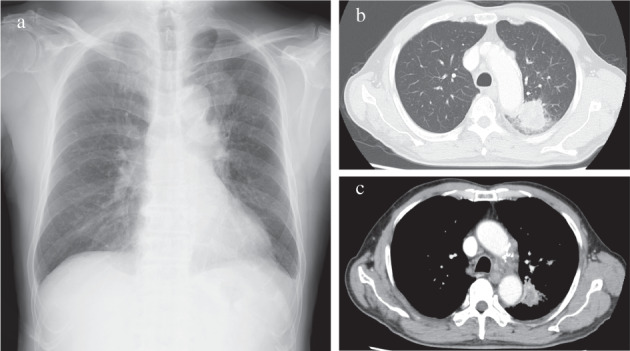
(a) Chest X‐ray before treatment. (b, c) Chest computed tomography (CT) before treatment. A 35 mm tumor was detected in the left upper lobe
